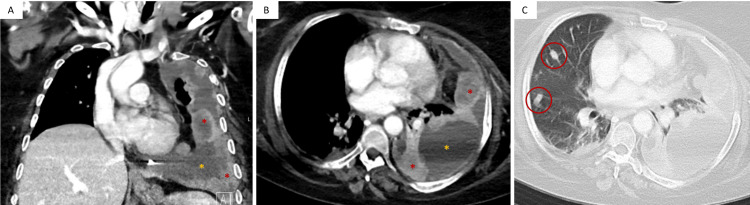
Contrast-enhanced CT scan of the chest revealing a large left-sided pleural mass consistent with a hemangiopericytoma/solitary fibrous tumor. Combined sagittal (A) and axial (B) views in the mediastinal setting and axial (C) view in the lung setting of the chest CT scan, showing a complete opacification of the left hemithorax with a contralateral mediastinal shift. The CT scan also showed extensive (>10 cm) subpleural soft tissue lesions in the left hemithorax (red asterisk) together with a significant amount of pleural fluid (yellow asterisk) and a complete atelectasis of the left lung, as well as multiple lung nodules (red circle) on the right side.
